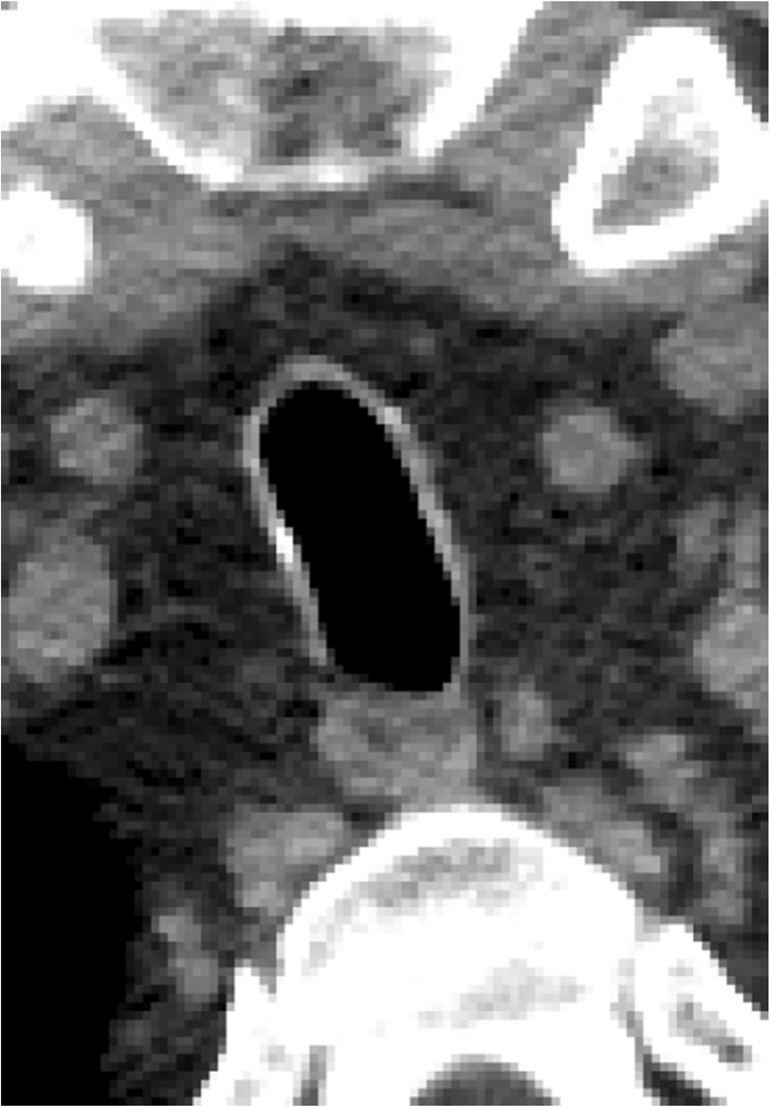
Sixty-two-year-old man with history of COPD. Axial CT in soft tissue window demonstrating intrathoracic saber sheath trachea, with a reduction in the coronal diameter of the trachea relative to the sagittal diameter
